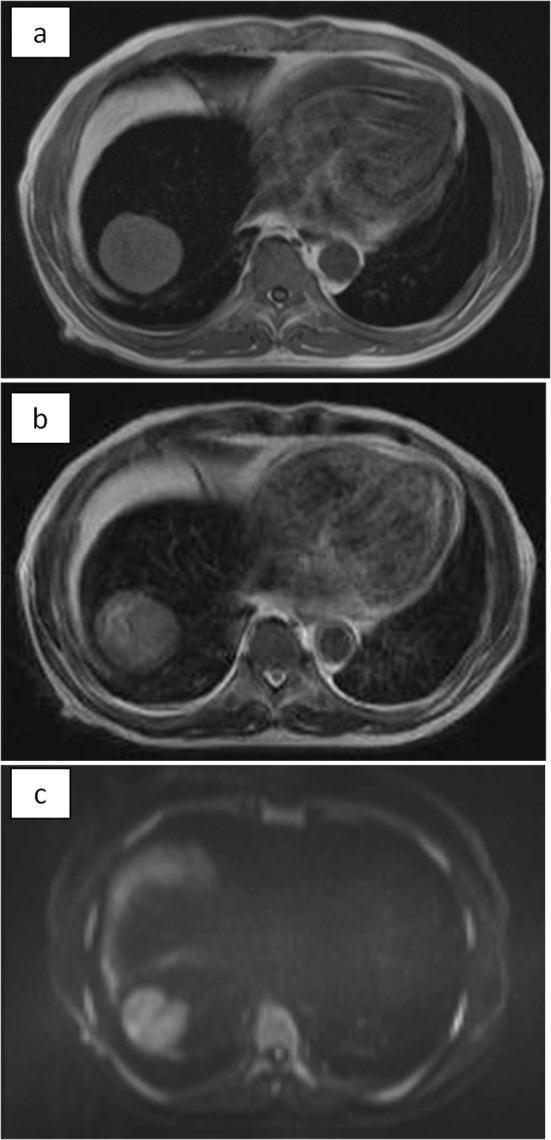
MRI. The tumor showed high intensity on T1 (a), T2 (b), and diffusion-weighted (c) images. The non-tumorous area of the liver showed prominently low intensities both on T1 and T2, indicating extensive iron deposition in the liver parenchyma. On diffusion-weighted image, the parenchyma also showed low intensity due to T2-blackout effect.
